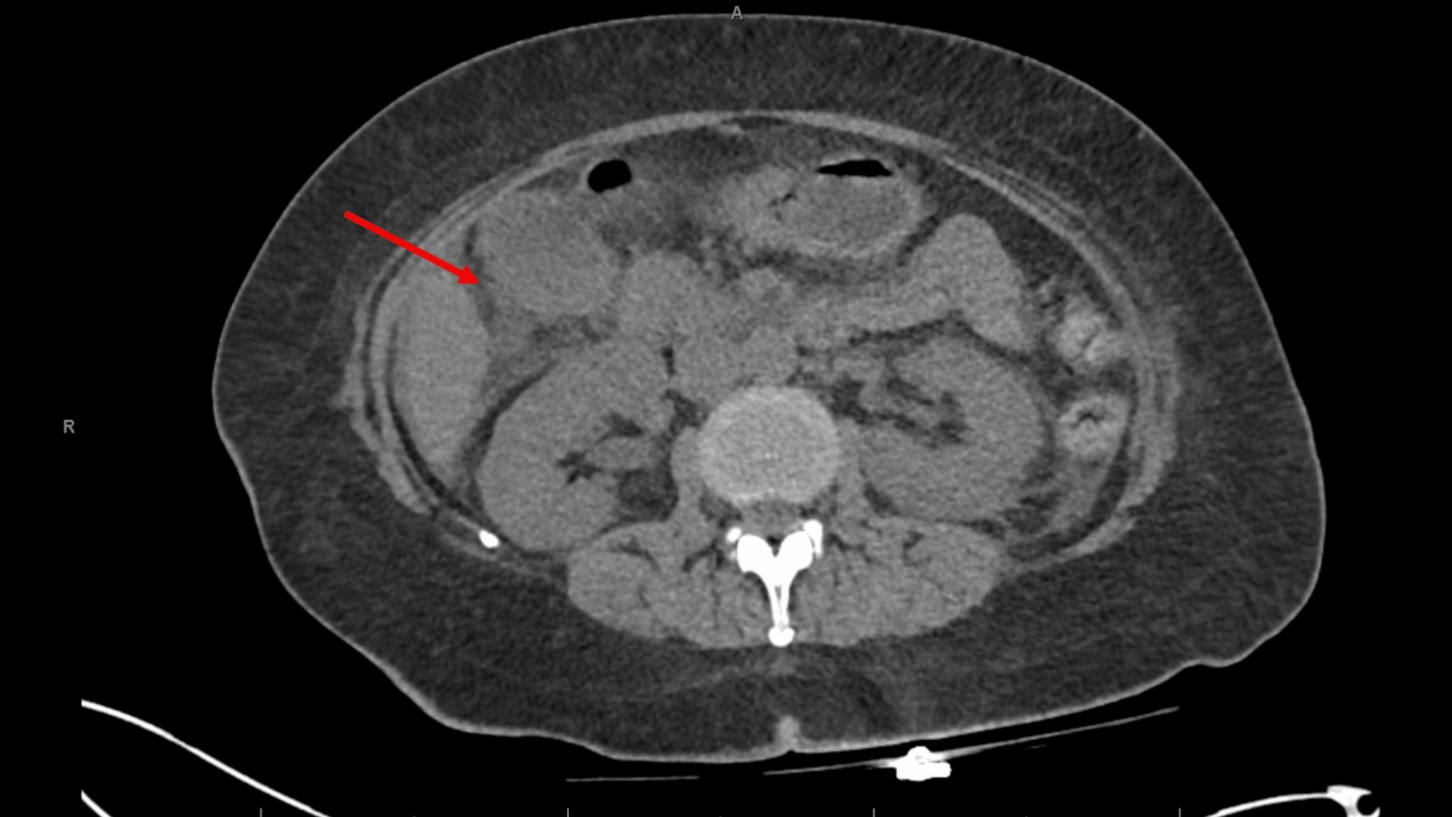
CT of the abdomen and pelvis CT imaging demonstrates gallbladder wall thickening with pericholecystic inflammatory stranding and fluid, findings concerning for acute cholecystitis. Small-volume ascites is also noted. CT, computed tomography
